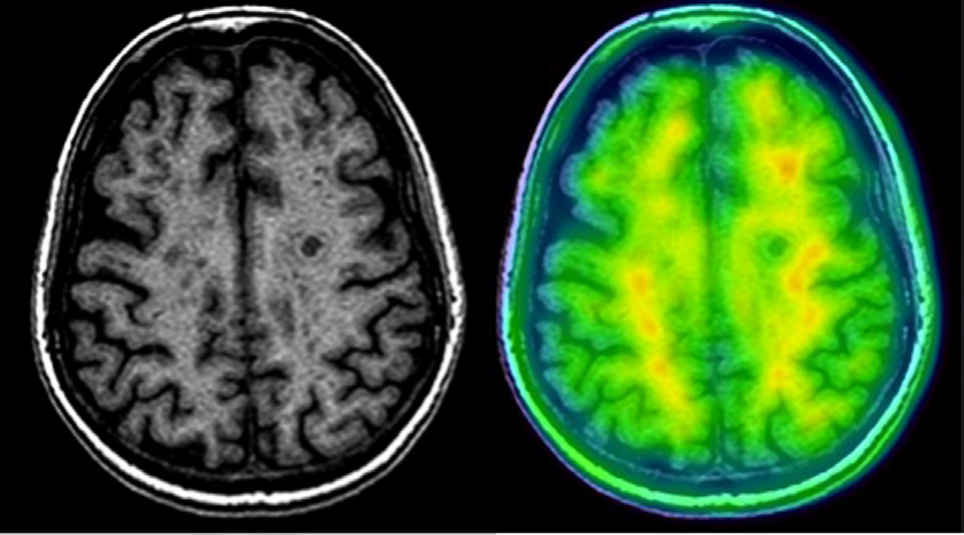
Amyloid-PET and MRI image of a patient with RRMS using ^18^F-florbetaben. Note the decreased uptake of the tracer in white matter lesions.
